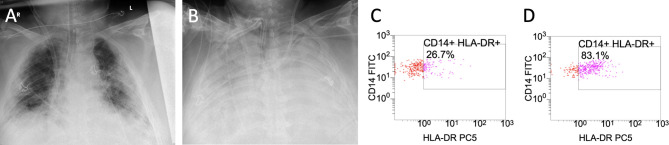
Caption shows the x-ray findings of the patient’s lungs on day 12 (A) and on day 28 (B). Flow-cytometry (FACS) results of monocytic human leukocyte antigen receptors (mHLA-DR) on monocyte (CD 14+) before administration of interferon-y (IFNy) on day 26 (C) and after interferon-y (IFNy) treatment on day 29 (D).
